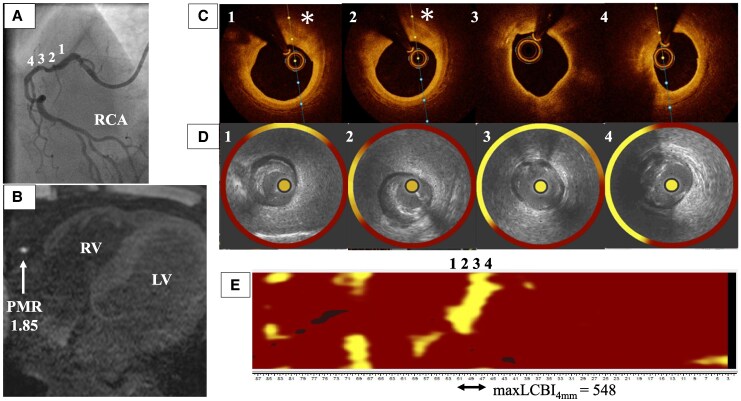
A representative case with target lesion exhibiting PMR ≥ 1.0. A 65-year-old gentleman was hospitalized due to angina pectoris. (*A*) Coronary angiography identified one significant stenosis at the middle segment of his RCA. (*B*) Non-contrast T1 imaging on CMR revealed that PMR at the corresponding lesion was 1.85 (white arrow). (*C*) OCT imaging prior to PCI visualized LIA (asterisks), accompanied by macrophage and cholesterol crystal. (*D* and *E*) PAV on IVUS imaging was 76.2%, and maxLCBI_4mm_ at the corresponding lesion was 548. CMR, cardiac magnetic resonance; IVUS, intravascular ultrasound, maxLCBI_4mm_; OCT, optical coherence tomography; PAV, percent atheroma volume; PMR, plaque-to-myocardium ratio.
